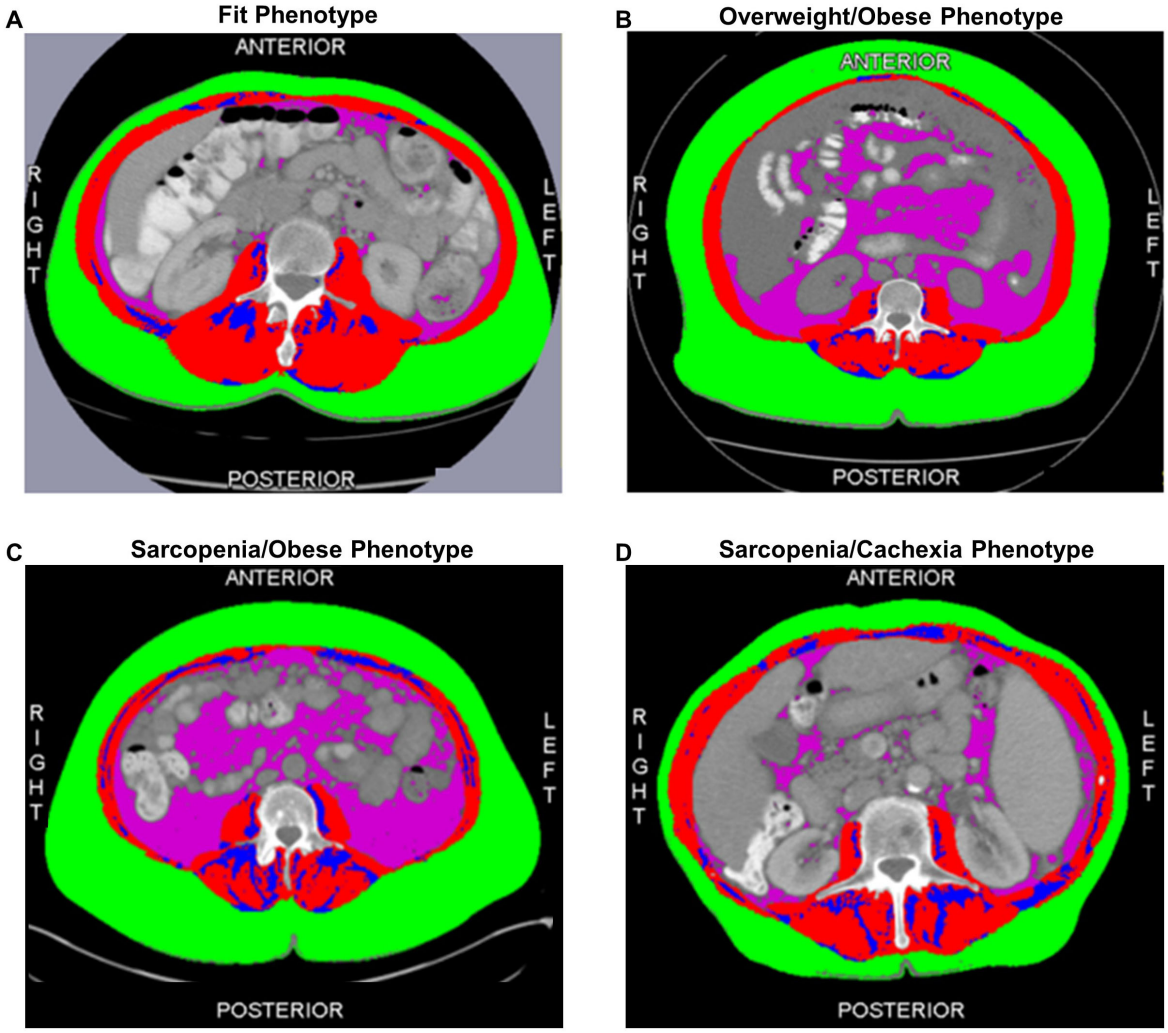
Cross-sectional computed tomography images at the third lumber vertebra depicting the adiposity and muscle distribution of each of the four body composition phenotypes including the (A) Fit (normal muscle/low total adiposity) Phenotype; (B) Overweight/Obese (normal muscle/high total adiposity) Phenotype; (C) Sarcopenia/Obese (low muscle/high total adiposity) Phenotype; and (D) Sarcopenia/Cachexia (low muscle/low total adiposity) Phenotype. The red compartment represents skeletal muscle; the green compartment represents subcutaneous adipose tissue; the blue compartment represents intermuscular adipose tissue; and the pink compartment represents visceral adipose tissue. Total adiposity is derived as the sum of the green, blue, and pink compartments.
